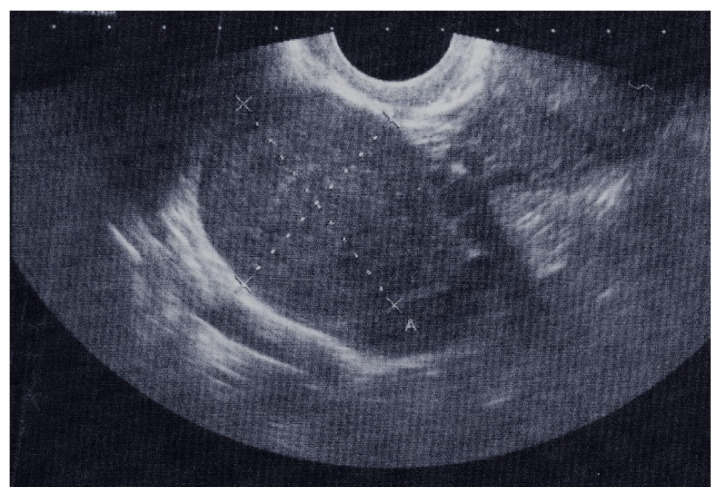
Transrectal sonography (TRS): solid tumor formation, 41.2 mm (A-X) × 29.5 mm (X-X) in diameter, with normal ovarian tissue on the periphery.
